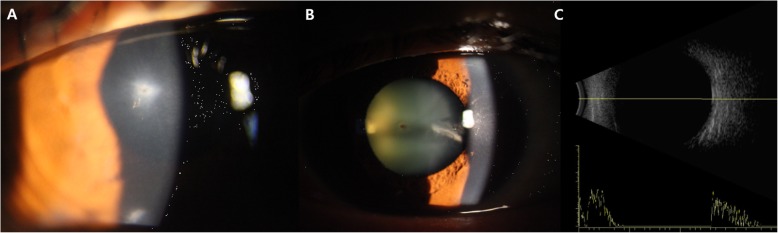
Anterior-segment photographs and B-scan sonography image at initial clinical presentation. a: Central corneal full-thickness laceration and little anterior chamber reaction at initial presentation. b: Anterior lens capsular rupture with a suspected foreign body. c: No evidence of intraocular foreign bodies or vitreous opacities on B-scan sonography
